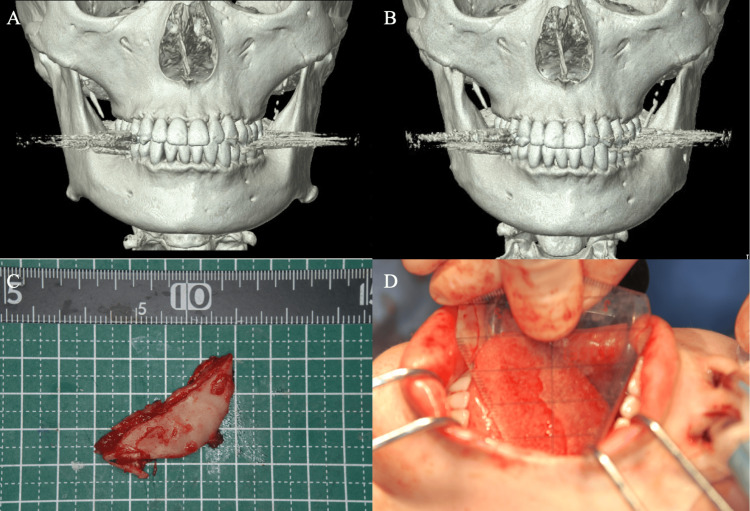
Mandibular anglectomy (A) Preoperative 3D CT image. (B) Postoperative 3D CT image. (C, D) Resection of the mandibular angle. The mandibular angle was resected equally on both sides using an oscillating saw blade. Intraoperative mouth opening change: from 40 mm to 60 mm.
